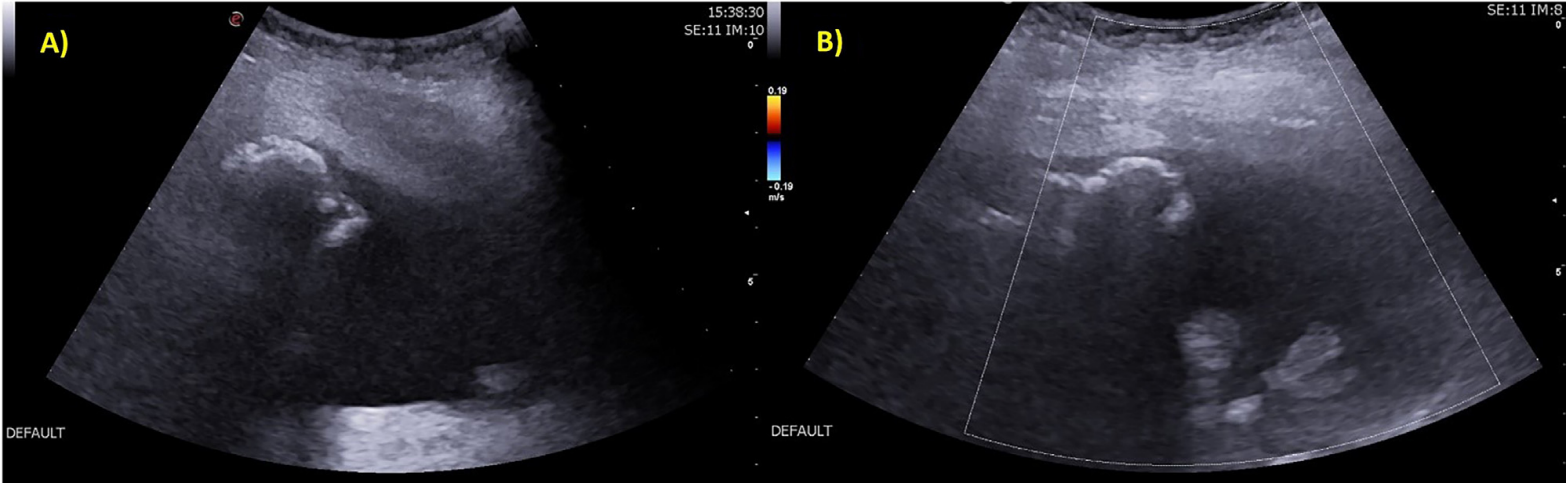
(A, B) US performed in orthostasis with the probe positioned at the posterior portion of the thigh, in the painful area reported by the patient. US in B-mode (A) showed a heterogeneous lesion with different types of content, with margins that were not definable. At Doppler application (B) there was no intralesional vascular signal.
